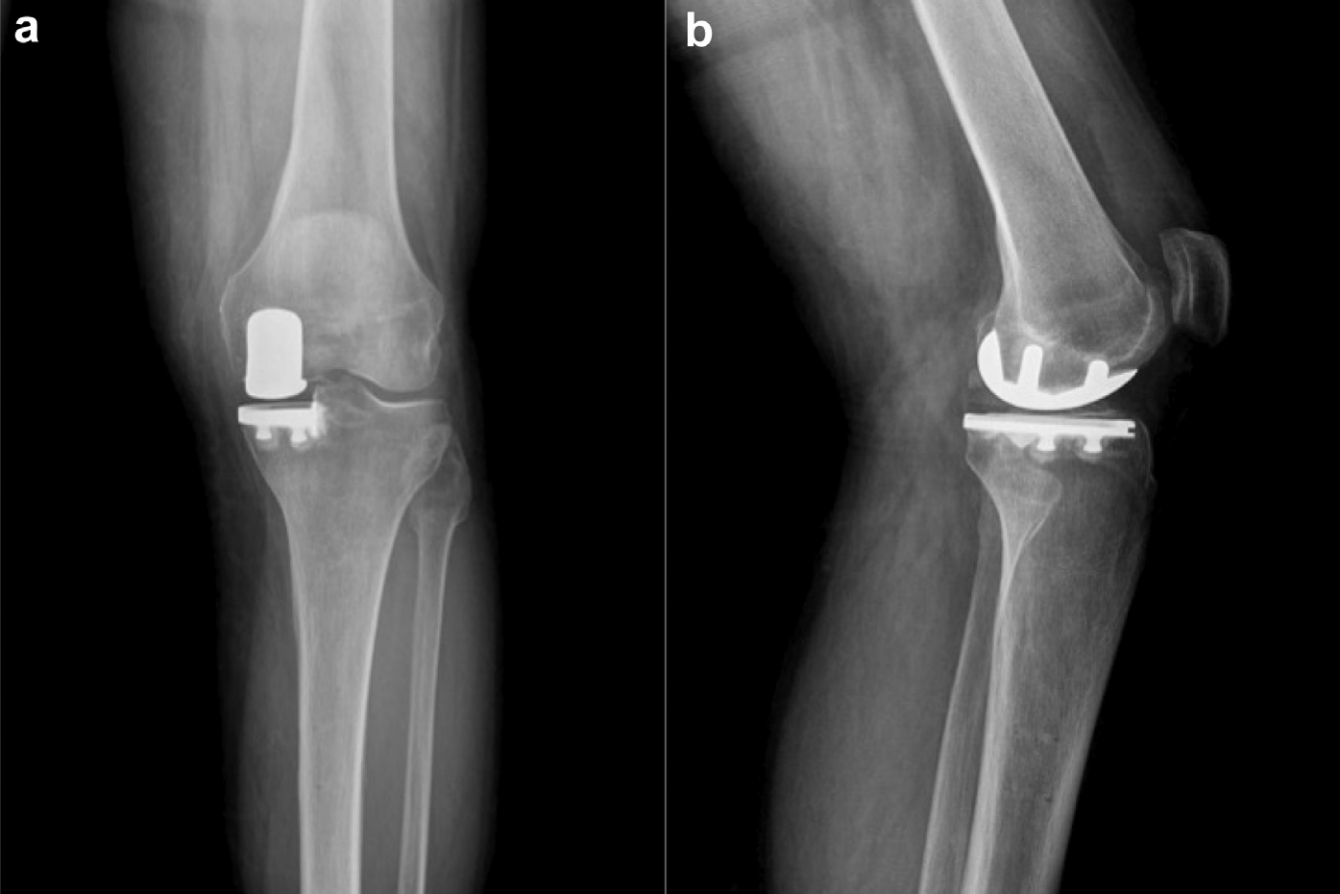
(a) Anteroposterior and (b) lateral radiographs of a study participant after receiving a partial knee replacement at their 8-year follow-up visit.
